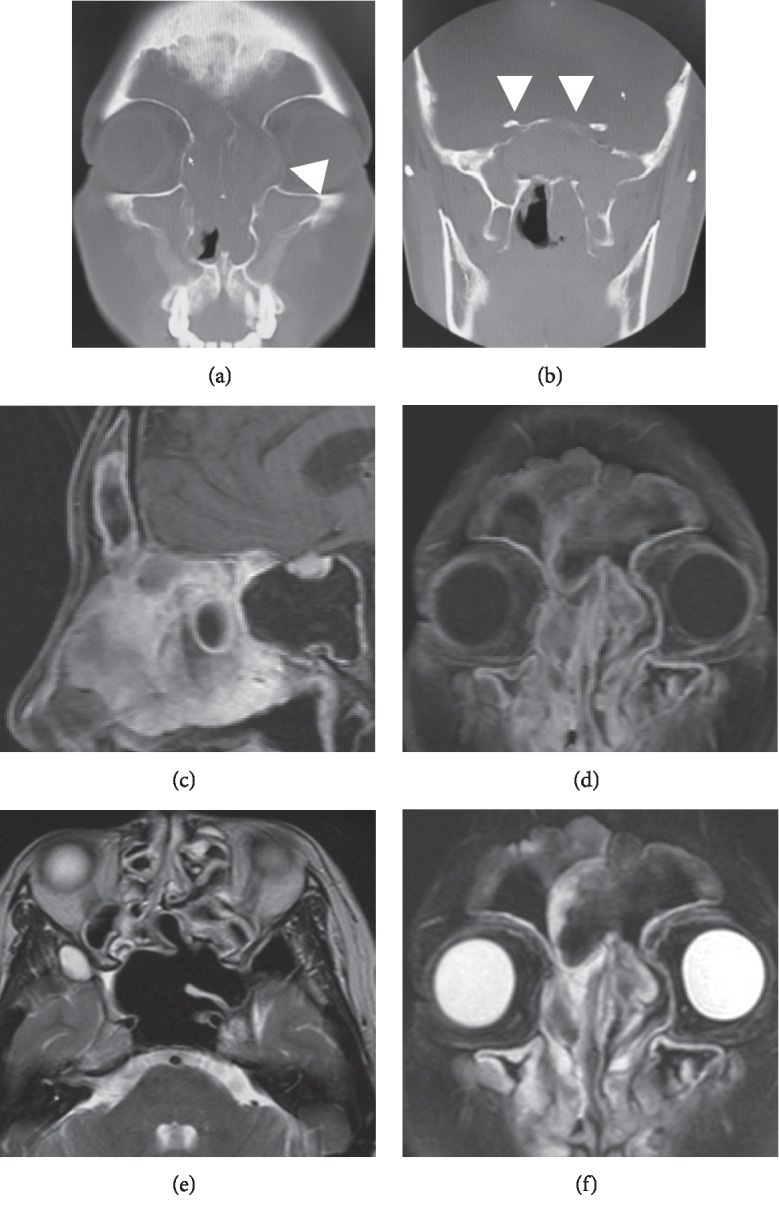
Image findings of Case 1 at the initial diagnosis. (a, b) Computed tomography images of the paranasal cavity (coronal section); bone thinning is evident (white arrow). (c) Gadolinium- (Gd-) enhanced T1-weighted MRI of the paranasal cavity (sagittal section). (d) Gd-enhanced T1-weighted image (coronal section). (e) T2-weighted image (axial section). (f) T2-weighted image (coronal section). Slightly high signals are evident at the paranasal sinus mucosa or nasal mucosa in the T1-weighted images, and a robust enhancing effect is evident in the Gd-enhanced image. Nonsignal regions are evident at the frontal, ethmoid, and sphenoid sinuses, along with an area showing low- to faint-high signal, indicating the possibility of allergic mucin in the nasal cavity. In the T2-weighted image, the paranasal sinus mucosa or nasal mucosa shows high signal levels, and most of the area with possible allergic mucin shows a low signal level.
